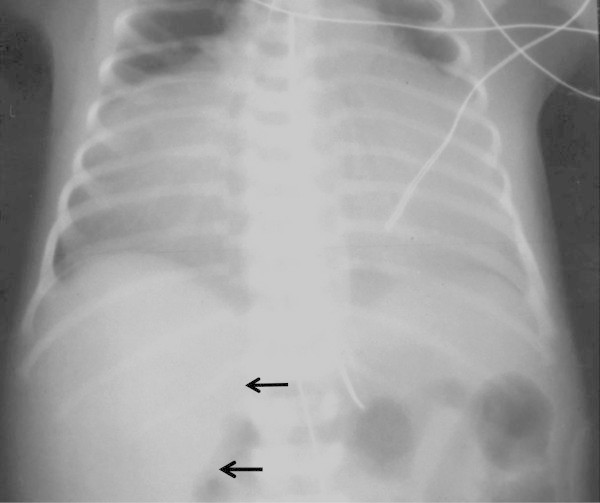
Chest radiograph 24 hours after repair of Bochdalek hernia. The grossly widened mediastinum, consisting of the mass and the heart, occupies almost the entire thorax. Only the right lobe of the liver is seen in the abdomen (marked by black arrows). The nasogastric tube and umbilical artery catheter are in place.
